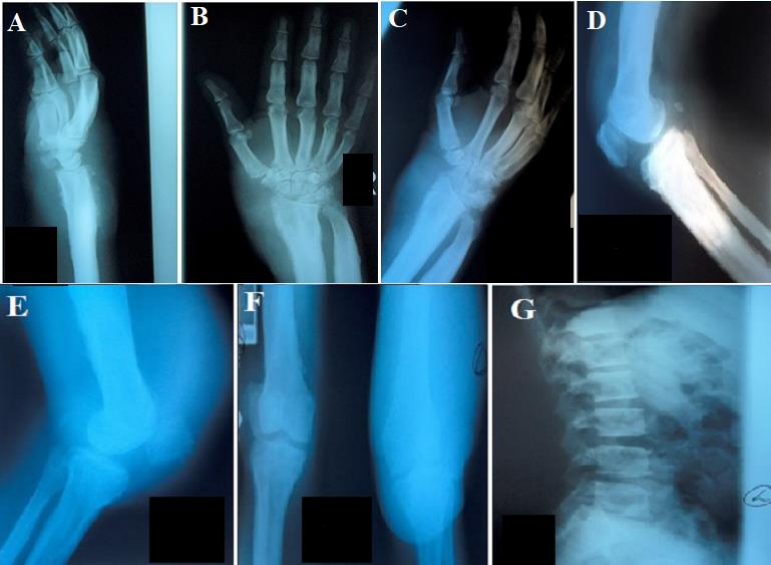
A, B, C) X-rays of both hands and wrists showing increased bone density and loss of corticomedullary differentiation, medullary expansion, and irregular periosteal reaction (spiculated), narrowing of joint spaces at radiocarpal joints associated with diffuse soft tissue swelling; D, E, F) X-rays of his knees showing spiculation around the patellae and proximal tibiae, with thickened cortex with medullary expansion; G) spinal X-ray showing increased bone density with mild degeneration of the spines with anterior osteophytes at L2-L5 and loss of lumbar lordosis
